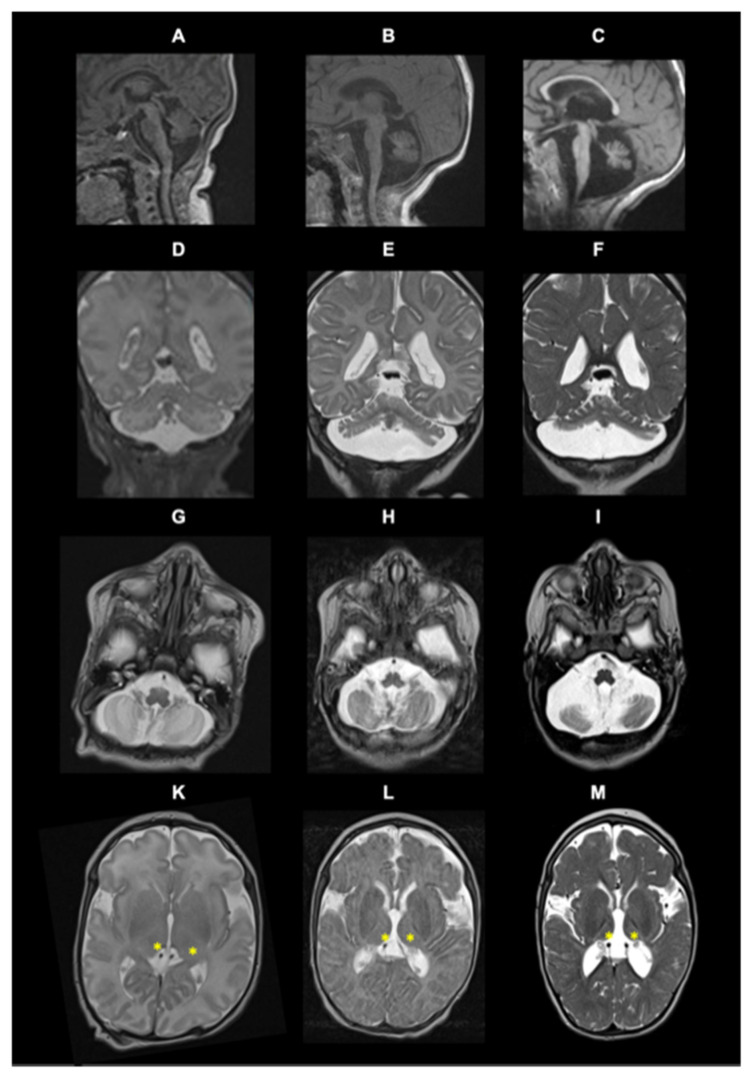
Brain MRI of the index case at the age of 2 weeks (A,D,G,K), 3 months (B,E,H,L), and 20 months (C,F,I,M). Description from first to last raw: sagittal T1 (A,B,C); coronal T2 (D,E,F); and axial T2 (G,K,H,L,I,M).
